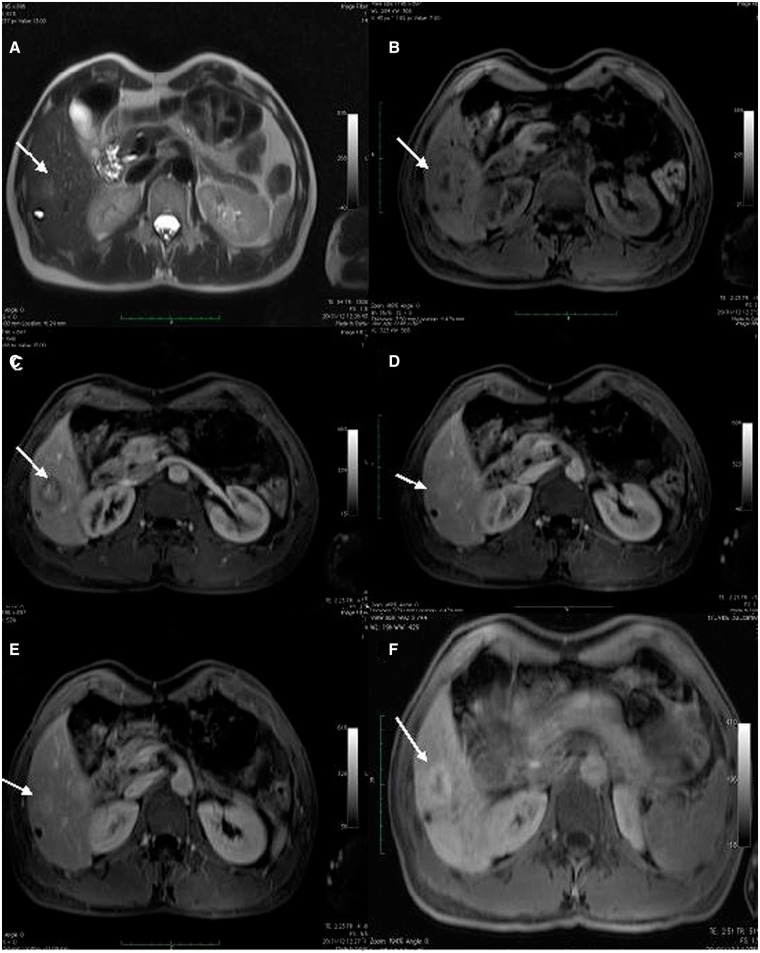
Contrast enhanced Magnetic Resonance Imaging (CEMRI), showing two focal liver lesions consistent with peliosis. T2-weighted (A), T1-weighted volume interpolated body examination (VIBE) (B), T1-weighted arterial phase (C), T1-weighted portal venous phase (D), T1-weighted late venous phase (E) and T1-weighted hepatobiliary phase (F) showing a focal liver lesion (arrows) slightly hyperintense on T2-weighted images with hypointense signal on T1-weighted images and centrifugal contrast enhancement and peripheral contrast media uptake on hepatobiliary phase.
